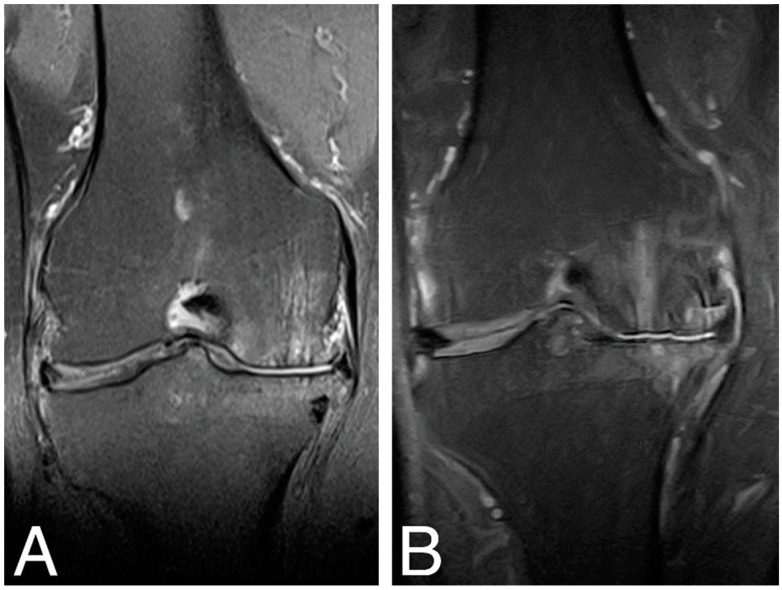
MRI images of the right knee, T2 sequence, and mid-joint frontal plane. (A) baseline (MOCART score 0.4); (B) 84 months time point (MOCART score 0.35).
